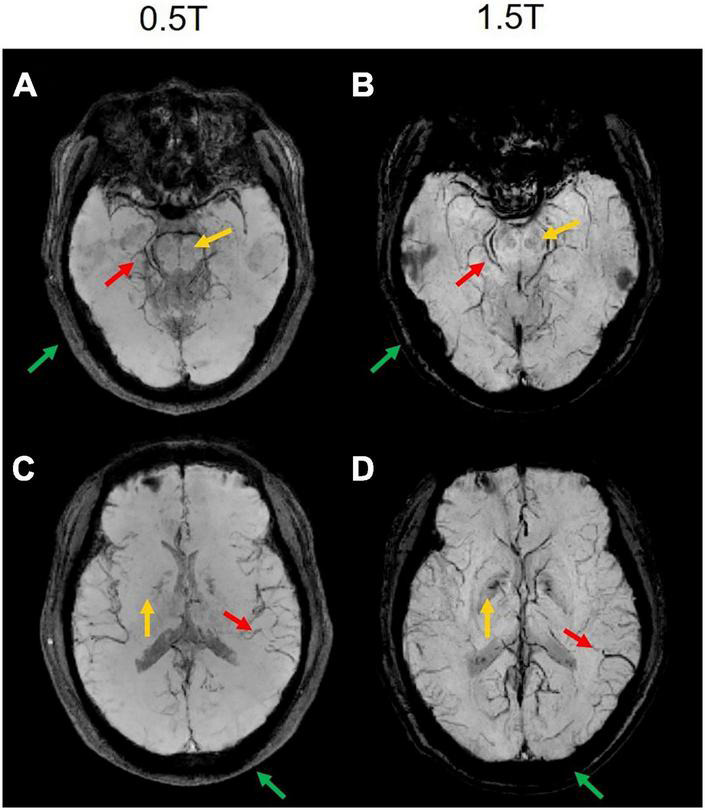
The comparison results of two representative slices from the same subject MRI scanning at 0.5T and 1.5T. (A,C) The magnitude-weighted susceptibility weighted images at 0.5T. (B,D) The magnitude-weighted susceptibility weighted images at 1.5T. Some small vessels are marked in red arrows, the red nucleus is marked in yellow arrows, the skull and extracranial lipid are marked in green arrows.
